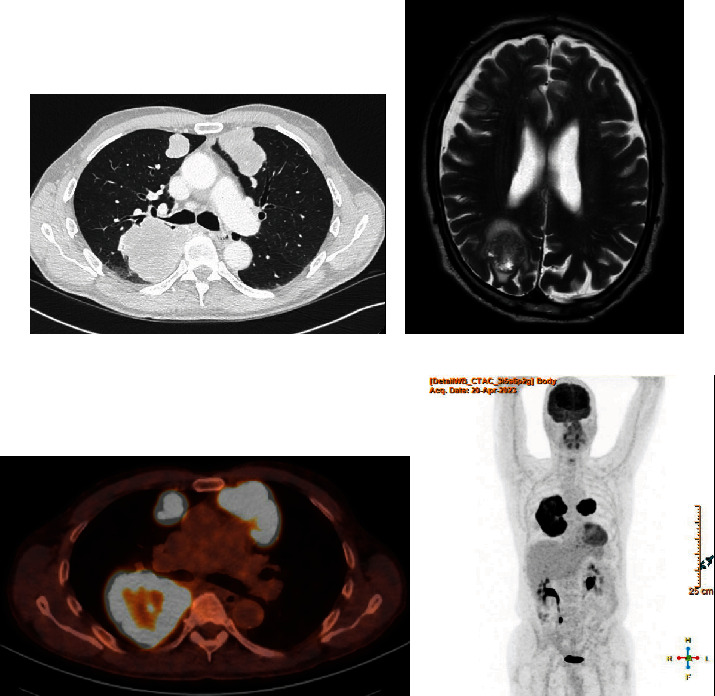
(a) A computed tomographic scan of the chest showing the 3 pulmonary masses with the largest mass located posteriorly in the right lower lobe. (b) MRI of the brain (T2-weighted axial image) showing the solitary brain metastasis consisting of a 2.7 cm nodular component with perilesional edema and hemorrhagic characteristics: susceptibility artifacts with foci of signal loss on T2-gradient echo sequence in the peripheral part of the nodule. (c, d) 18F-FDG PET/CT showing FDG avidity and extent of the bilateral pulmonary tumoral sites with physiologic uptake in the urinary tract.
